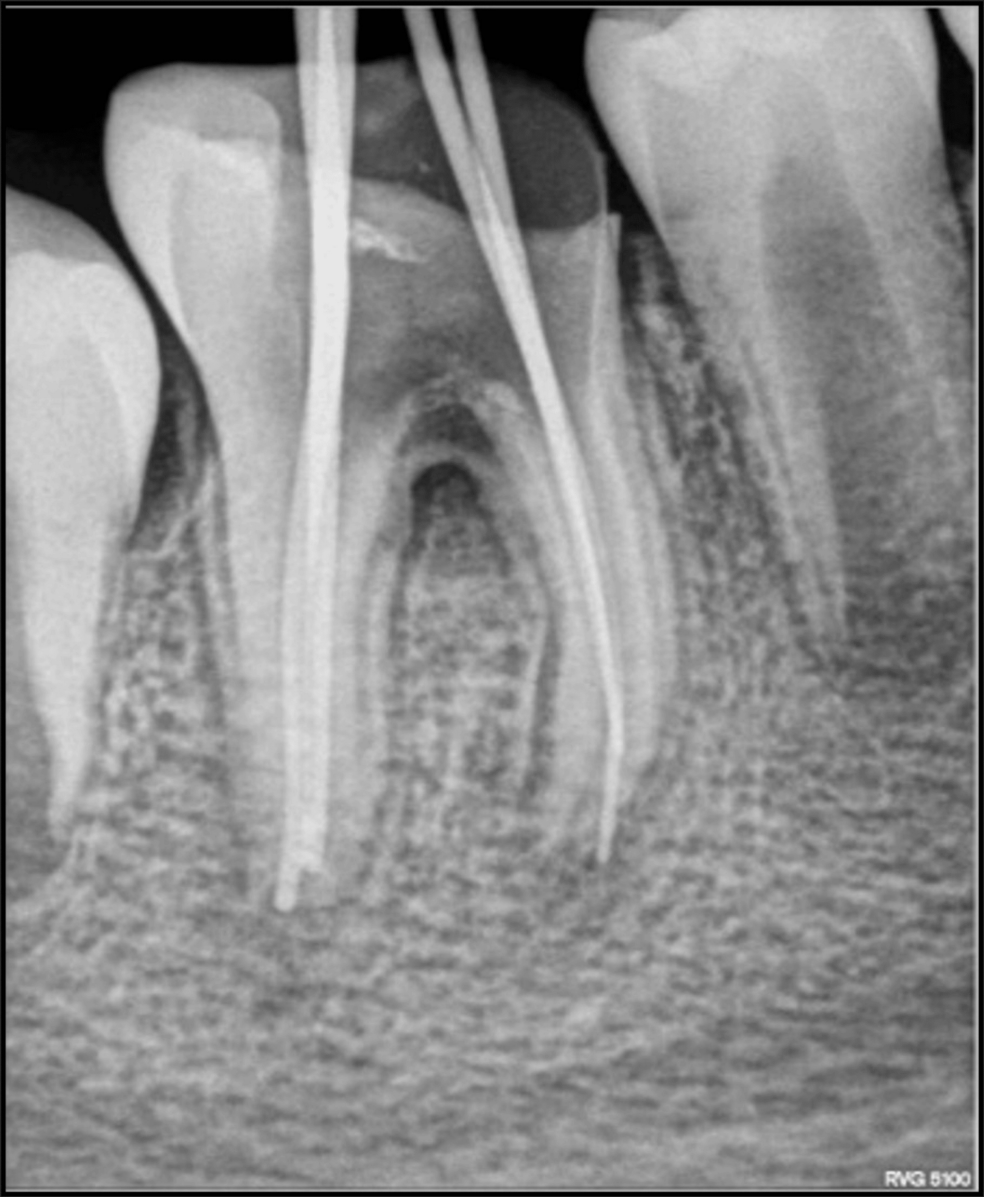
Intraoral periapical (IOPA) radiograph to check mastercone with respect to 46
